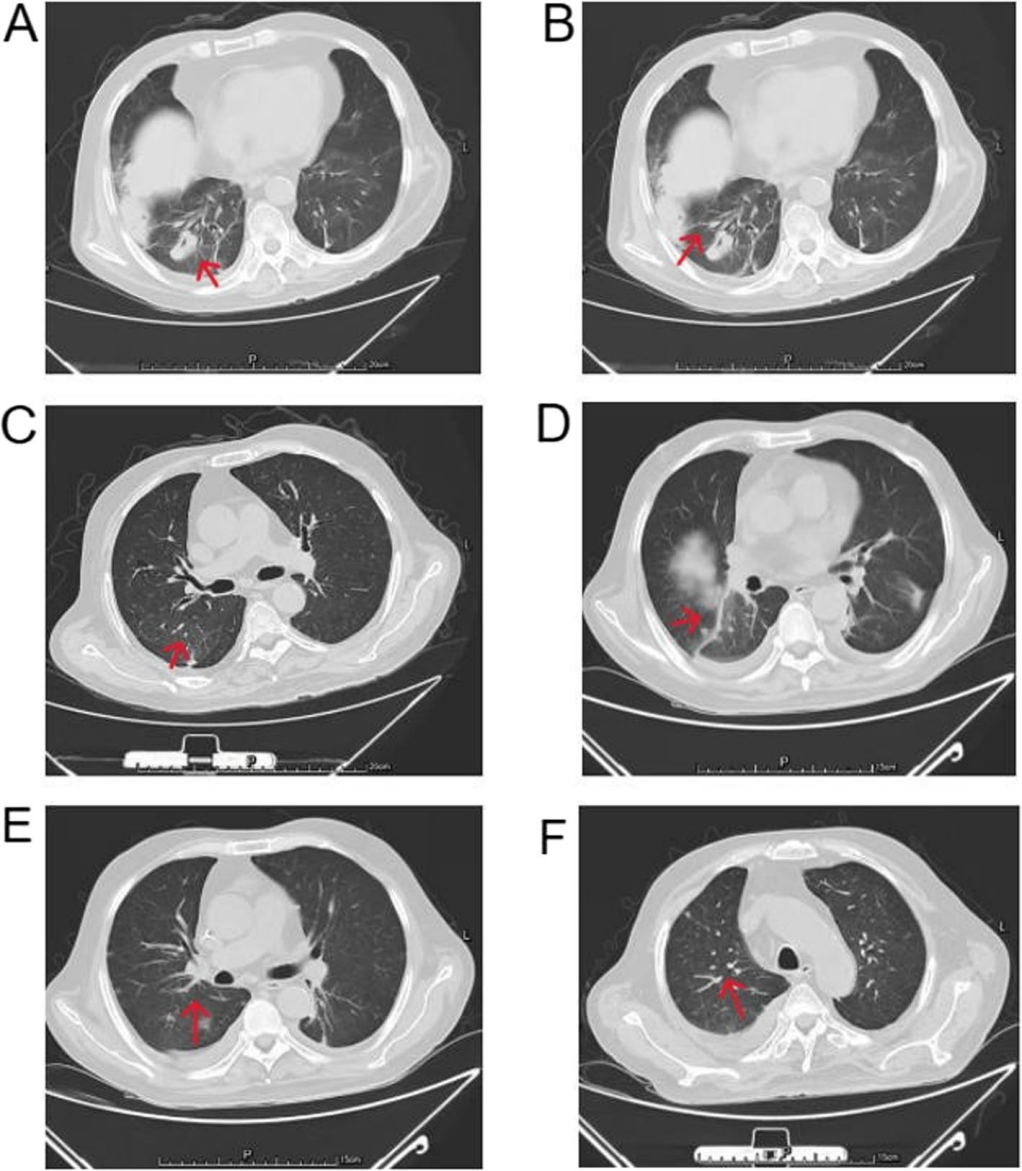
A-C Admission chest CT displayed interstitial changes in both lungs, a small amount of effusion in the right thoracic cavity, multiple small nodular and strip-like dense shadows. D-F Two weeks after admission chest CT showed a significant reduction in patchy shadows in both lungs
